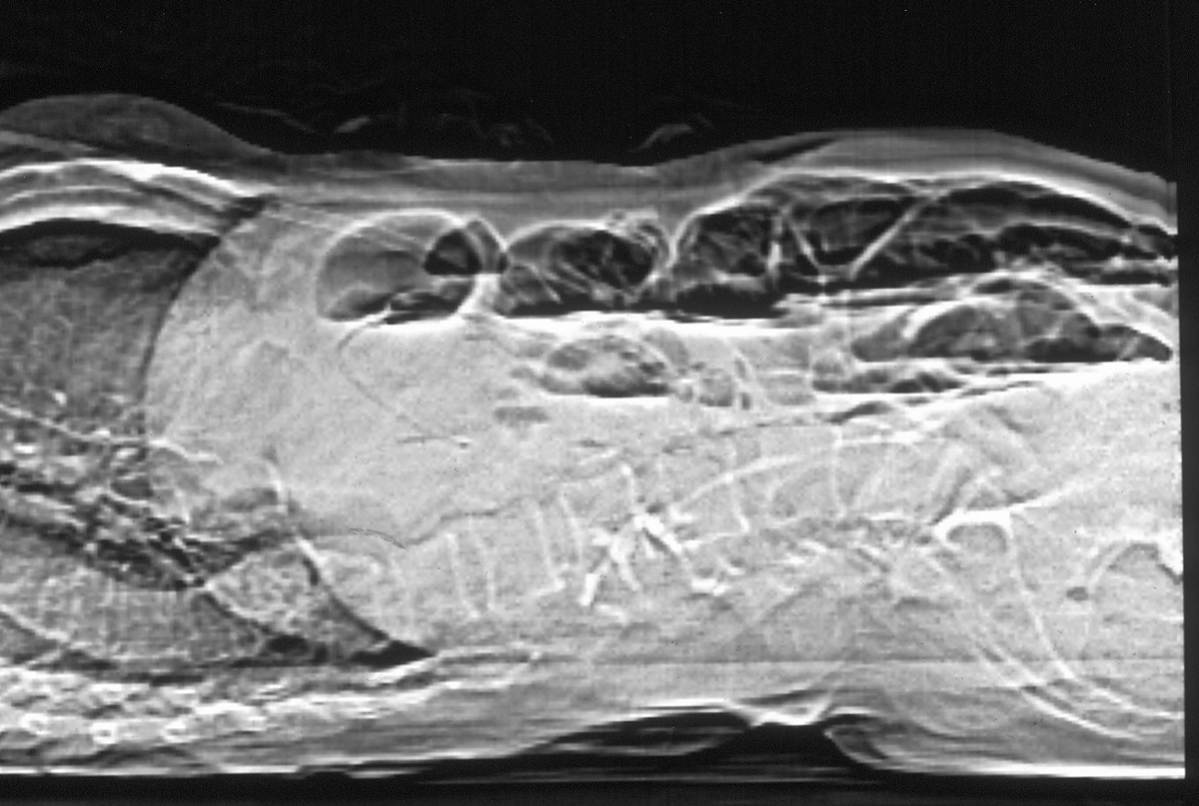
CT scan L-L: Marked hydro-gaseous distention of jejunum-ileal loops with multiples air-fluid levels.
